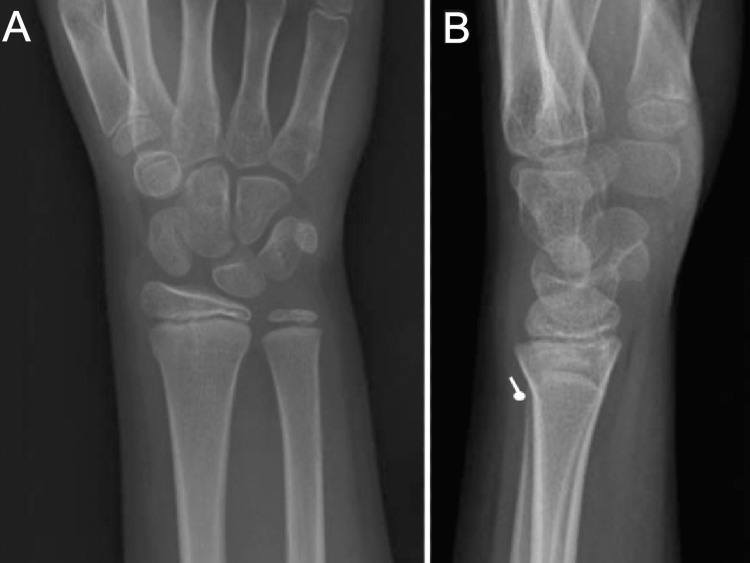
Angled buckle fracture. Posterior-to-anterior (A) and lateral (B) X-rays of a right wrist in a skeletally immature individual demonstrating an angulated DRBF. (B) Note the existence of a single cortical deflection, as is typical of an angled DRBF. Images courtesy of The Radswiki, Radiopaedia.org, rID: 12025 [11]. Note: this image was digitally modified to appear more like an angled DRBF because the original could be mistaken for a Salter-Harris. DRBF: distal radius buckle fracture
